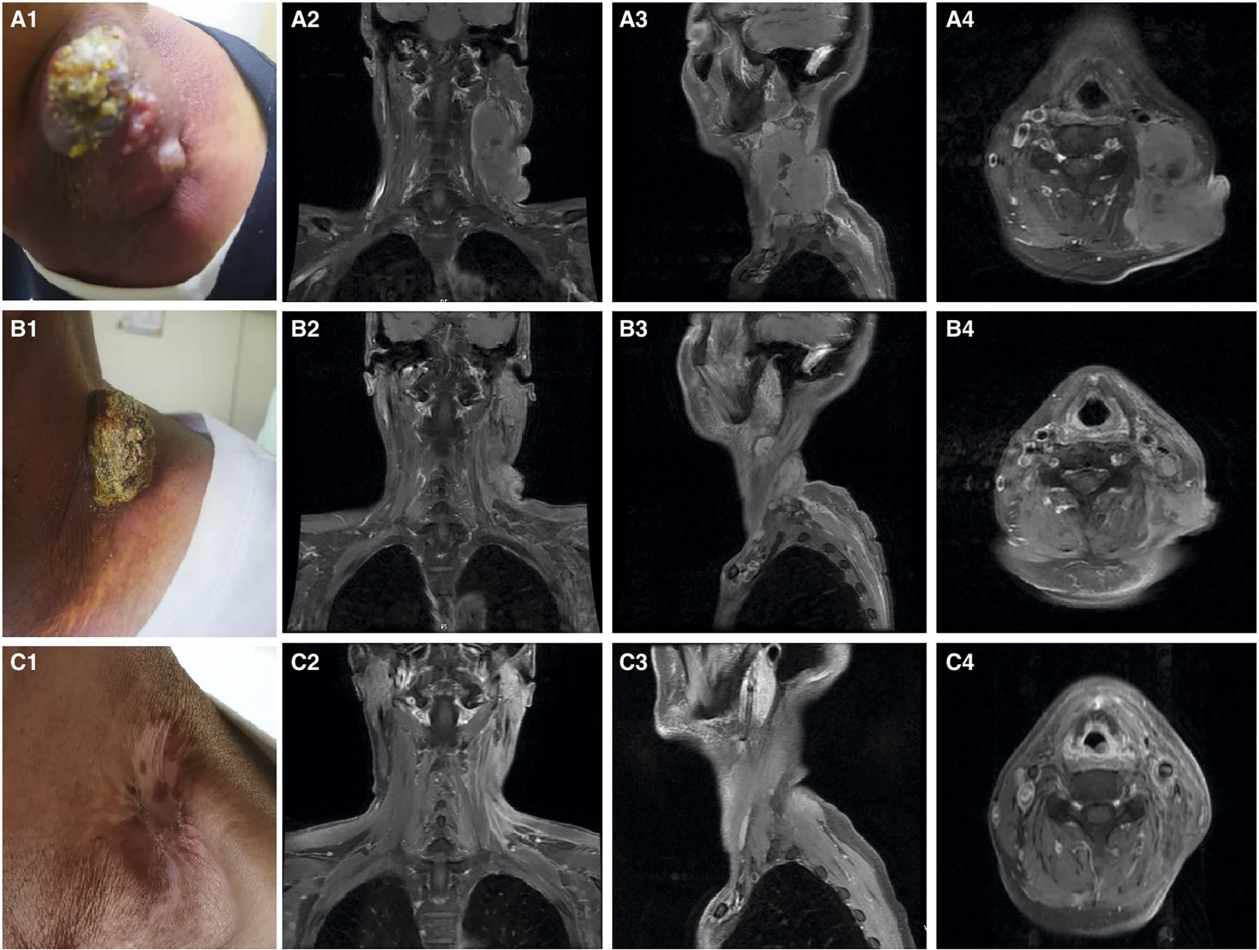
Imaging of the patient on treatment. (A1–A4) Pretreatment. (B1–B4) After chemoradiotherapy. (C1–C4) After 3 cycles of immunotherapy. (A1) Solid mass in the left neck. (A2–A4) MRI showing a large mass lesion in the left neck with liquefactive necrosis. (B1) Tumor is significantly reduced. (B2–B4) MRI evaluation. The left neck tumor is significantly reduced, but residual shadows are still visible. (C1) The neck mass disappears. (C2–C4) MRI evaluation. The left neck tumor and the lymph nodes under the armpit are not palpable.
